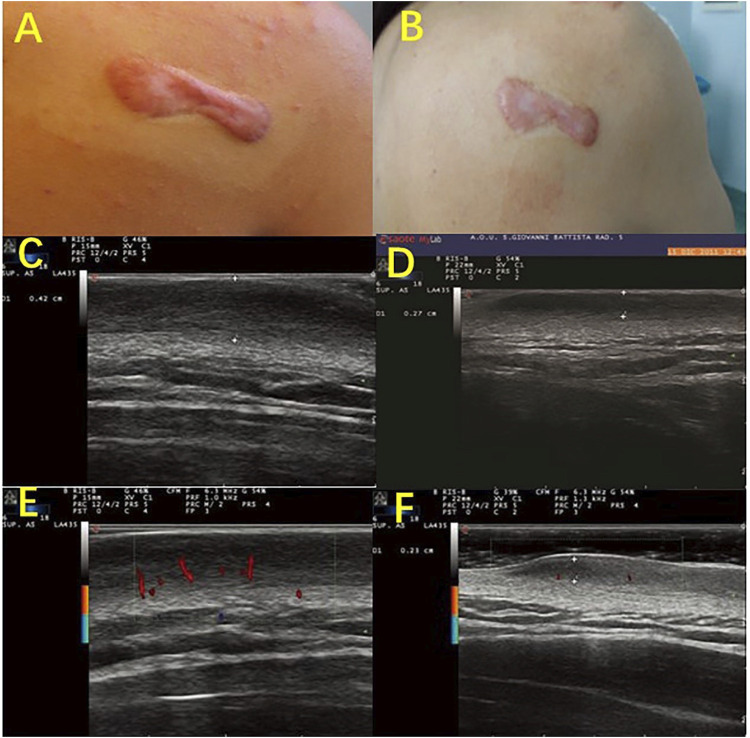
Color-Doppler ultrasonography evaluates the characteristic vasculature of scars. Photography of a scapular keloid before treatment (A) and after 1 month of treatment with a Pico device (B). B-mode Ultrasound images of the keloid before treatment (C), after 1 month of treatment (D), and after 2 months of treatment with a Pico device (F), show that the keloid thickness was reduced from 0.42 to 0.27 cm and then to 0.23 cm. Color-Doppler ultrasound images showed palisade vessels in the keloid before treatment €, and palisade vessels disappeared after 2 months of treatment with a Pico device (F).
